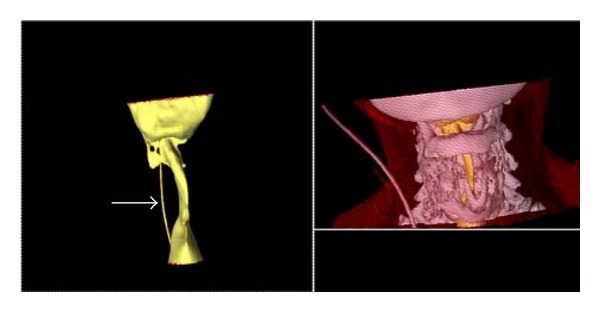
Three dimensional CT reconstruction of the larynx, yellow structures are air. Gray areas are bony structures. There is narrowing of the airway of 4 mm over a 4 cm long trajectory at the subglottic level. White arrow: reconstruction of air column in the feeding tube which is present in the oesophagus.
